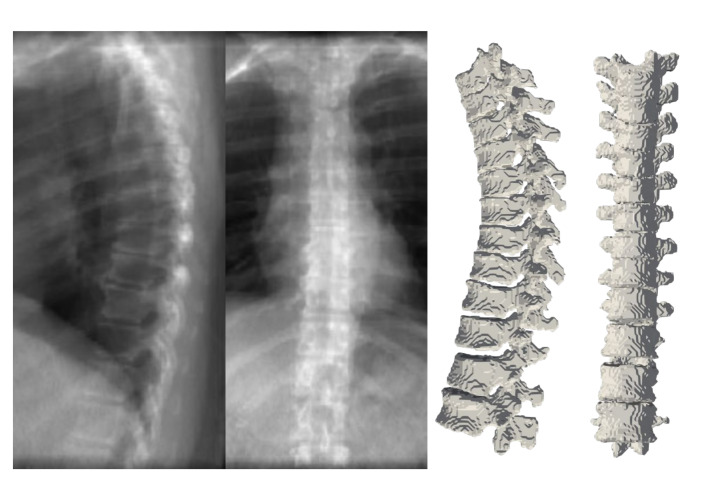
Orthogonal DRRs of a patient and reconstructed 3D spine model. DRRs are generated from CT scans and our model is trained to reconstruct the 3D spine shape from DRRs.
